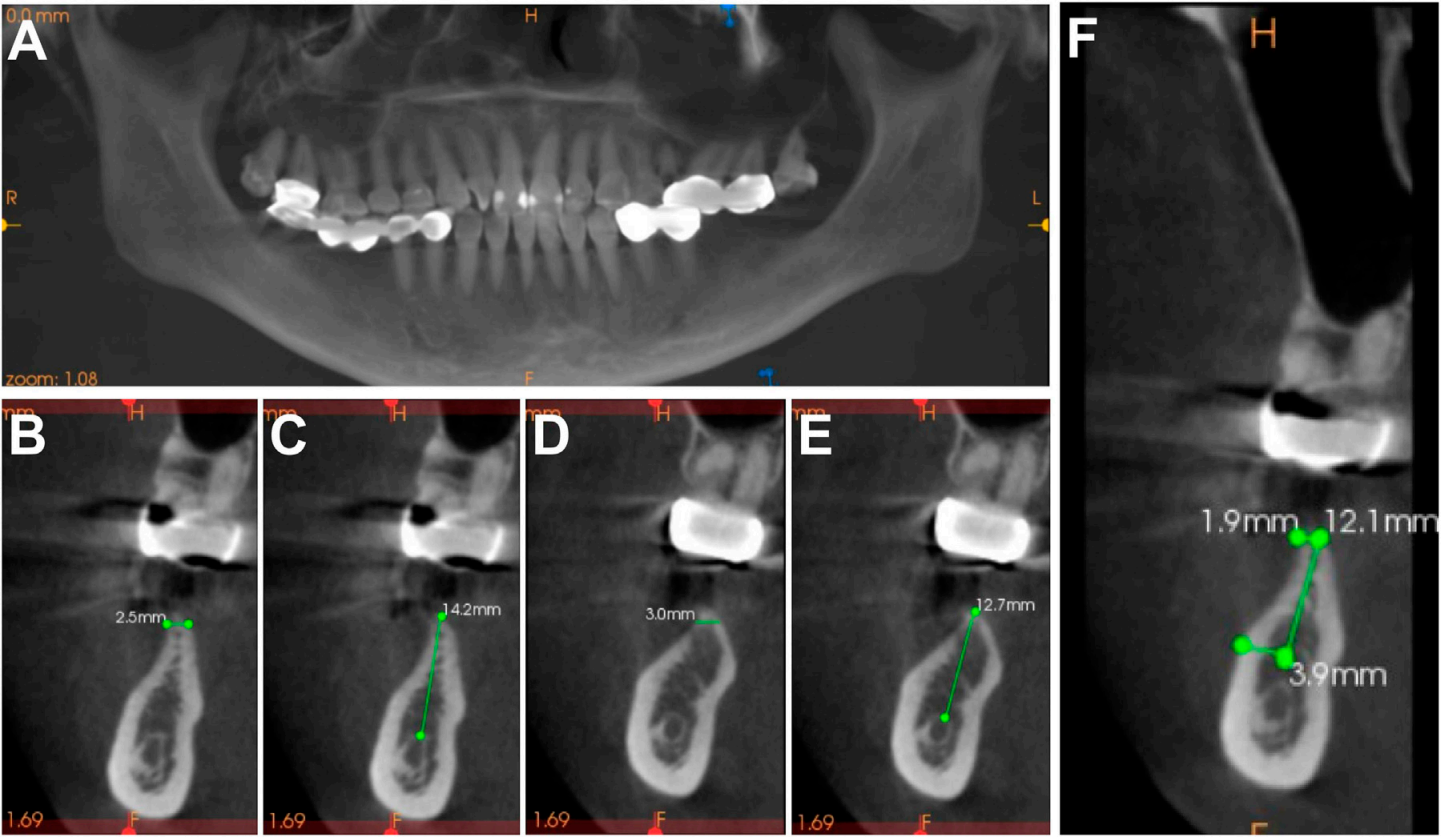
Pre-operative CBCT and surgical plan design (A) CBCT panoramic image. (B) Available bone height of implant sites #36. (C) Alveolar ridge width of implant sites #36 (taking the baseline 1 mm below the dental ridge crest). (D) Available bone height of implant sites #37. (E) Alveolar ridge width of implant sites #37 (taking the baseline 1 mm below the dental ridge crest). (F)
